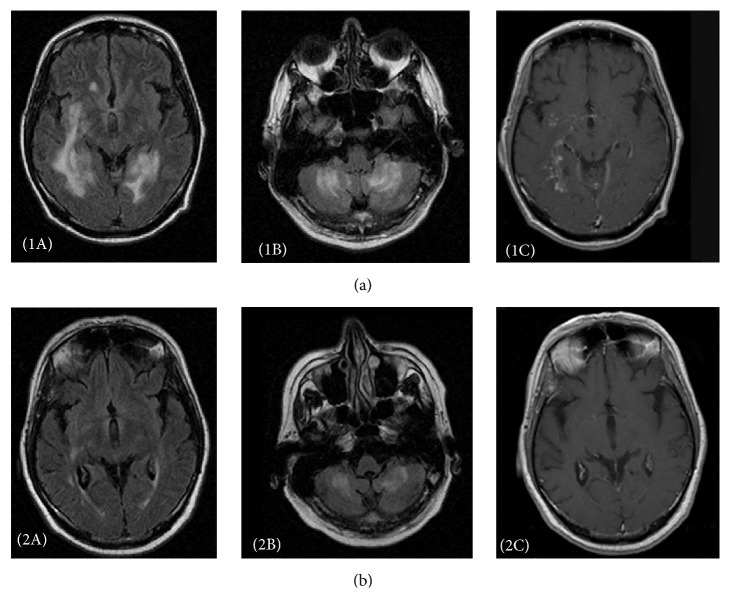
(a) Pretreatment aspects. T2 FLAIR sequences showing disseminated and confluent subcortical hyperintensities within frontoorbital, insular, and internal temporal regions (panel 1A) and cerebellum (panel 1B). Gadolinium injection (panel 1C) reveals nodular enhancement within the same regions and in leptomeningea. ((b) panels 2A, 2B, and 2C) Posttreatment aspects within approximately the same slices, showing an important regression of pathological aspects observed previously.
